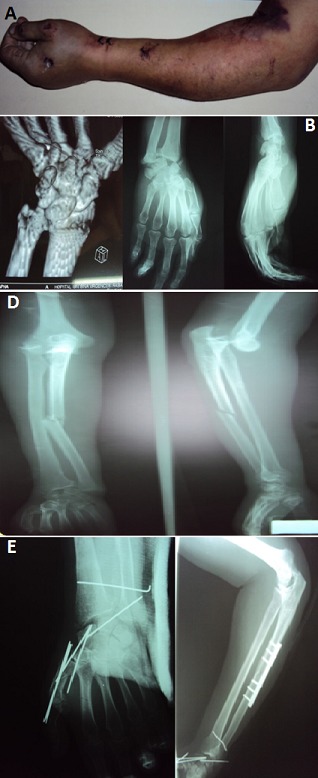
(A) avant-bras et main gauches œdématiés, coude et poignet déformés et ecchymotiques; (B) radiographie de la main gauche de face et de profil complétée par un scanner 3D confirmant la luxation périlunaire du carpe associée à la fracture transtubérositaire du scaphoïde type IV de Schernberg et à la fracture de la base du 1er métacarpien avec la déformation du poignet en Volarintercalated segmental instability; (C) radiographies de l'avant-bras gauche montrant les luxations postéro-externe du coude, de la radio-ulnaire distale et transcapho-périlunaire antérieure associées aux fractures de la diaphyse de l'ulna, de la styloïde radiale et de la base du 1er métacarpien; (D) radiographie de contrôle de l'avant-bras et de la main gauches montrant l'embrochage du scaphoïde, de la scapho-lunaire, de la radio-ulnaire distale et de la base du 1ermétacarpien et ostéosynthèse le l'ulna par plaque spécial radius après réduction de la luxation du coude
